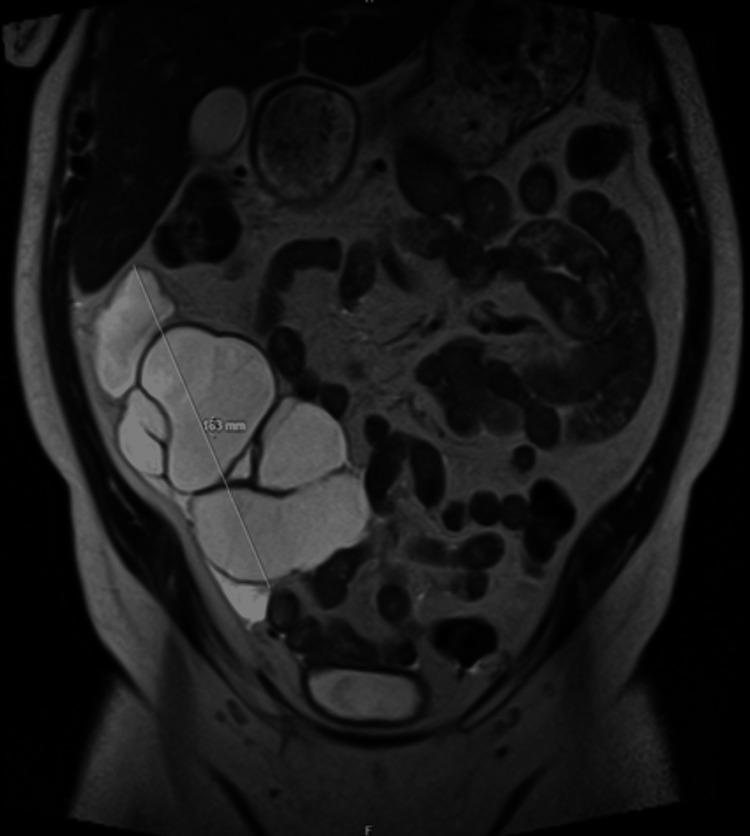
MRI showing large septated cystic lesion closely associated with the ascending colon and small bowel.
